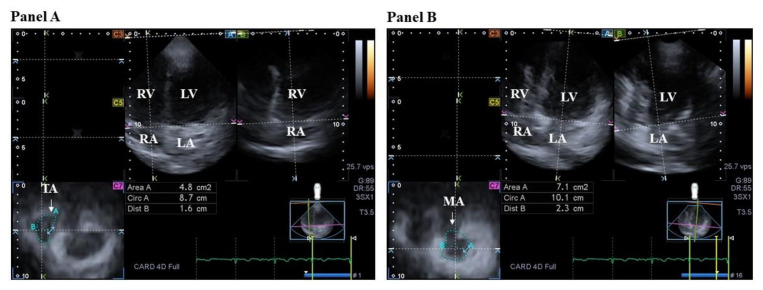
Three-dimensional (3D) speckle-tracking echocardiographic presentation of the two-dimensionally projected views of tricuspid (TA) (panel A) and mitral annuli (MA) (panel B). From the acquired 3D echocardiographic dataset, the following views can be created: apical four-chamber (A) and two-chamber views (B) and a cross-sectional view at the level of the TA/MA optimized in apical four- and two-chamber views (C7). The white arrow represents the TA/MA plane. Abbreviations: LA = left atrium, LV = left ventricle, RA = right atrium, RV = right ventricle, Area = TA/MA area, Circ = TA/MA perimeter, Dist = TA/MA diameter.
